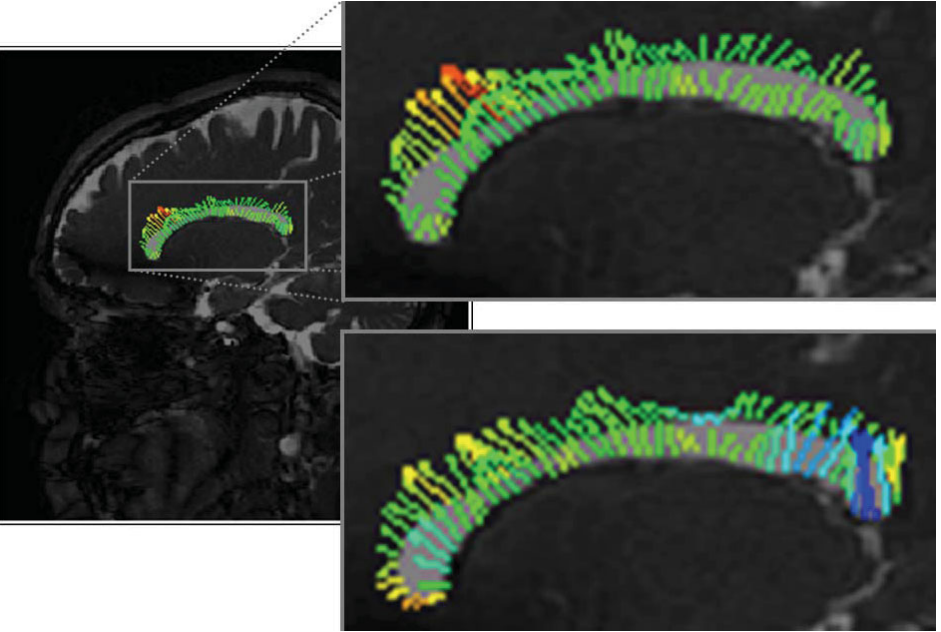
DPIV volume change estimation technique. Sagittal MR images at two different times, with Digital Particle Image Velocimetry (DPIV) derived displacement vectors along the lateral ventricle's walls. The vector lengths have been magnified for clarity. Vector color is based on wall normal displacement: red represents distension, blue contraction, and green nominal normal displacement.
